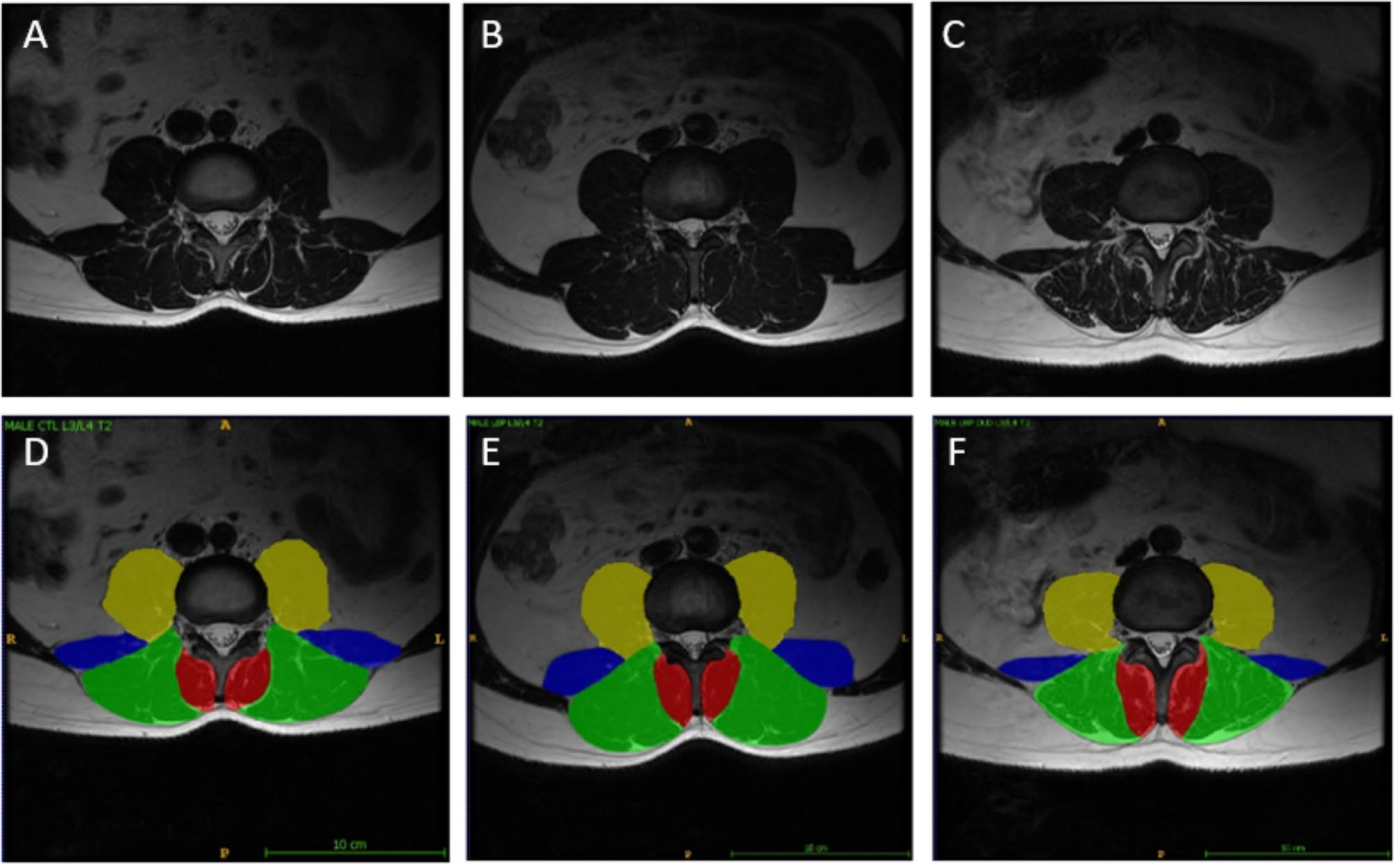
All images are T2-weighted images at the L3/L4 level in males. The first column (A & D) are images of the control. The middle column (B & E) are images of an age-matched individual with LBP. The third column (C & F) are images of the older individual with LBP. D, E, and F include the segmentations of the lumbar multifidus (red), erector spinae (green), quadratus lumborum (blue), and psoas (yellow) muscles
